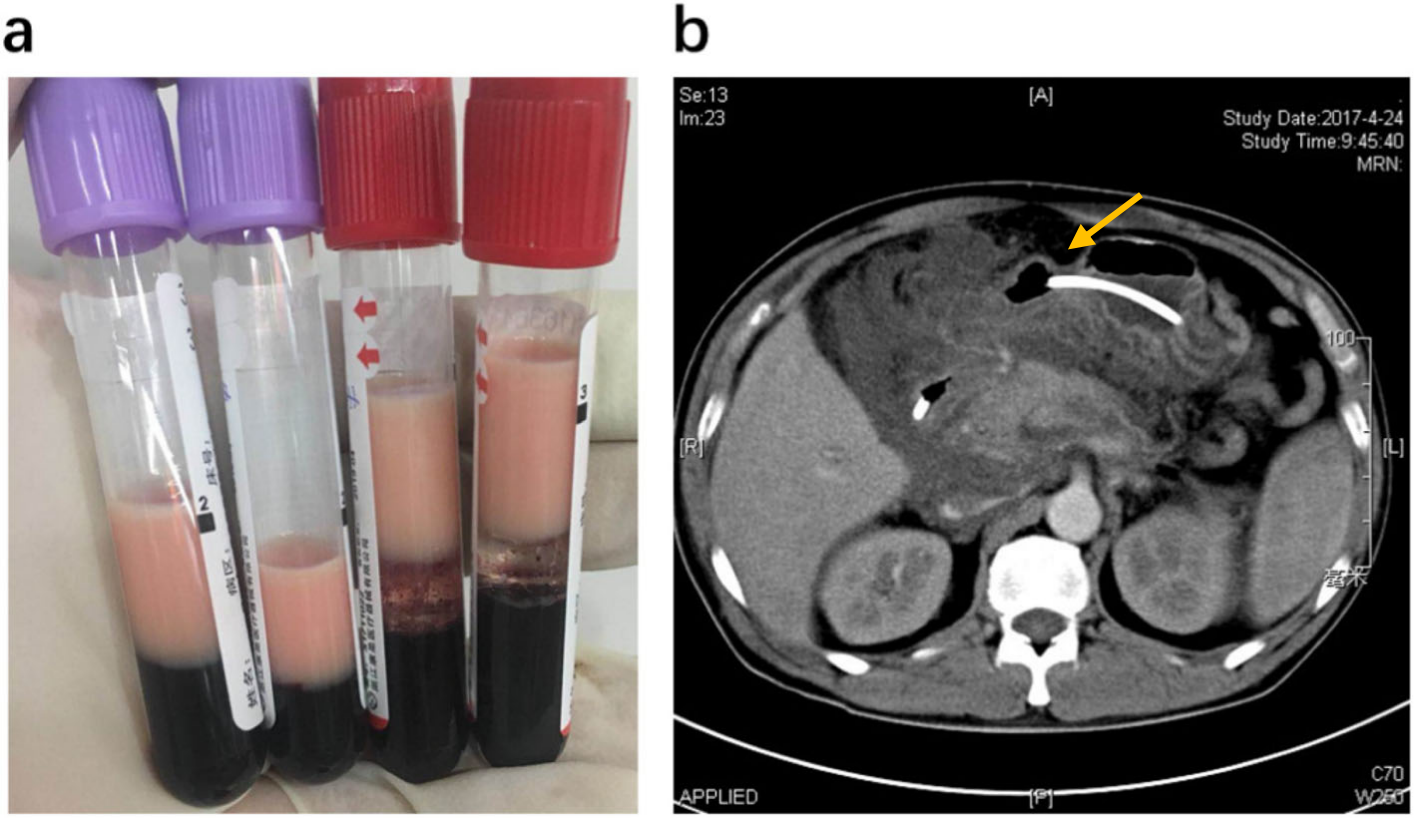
Two clinical observations of the patient upon admission to our service. a Blood samples showing chylemia. b Abdominal computed tomography showing enlarged pancreas with adjacent water density shadow and disappearance of the space between the pancreas and peripancreatic adipose tissues (arrow)
